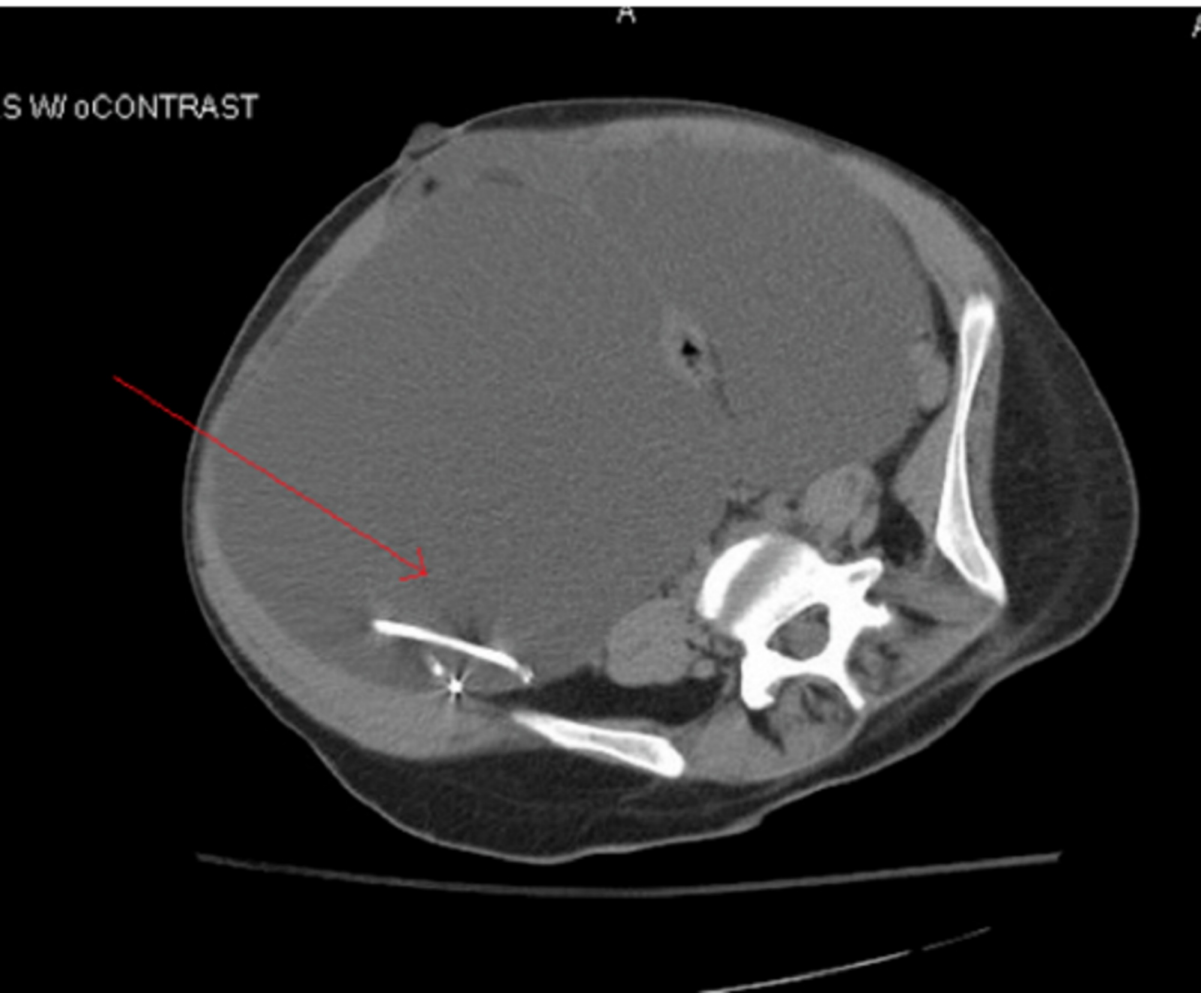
Axial CT showing abdominal fluid collection adjacent to ventriculoperitoneal shunt catheter tip
